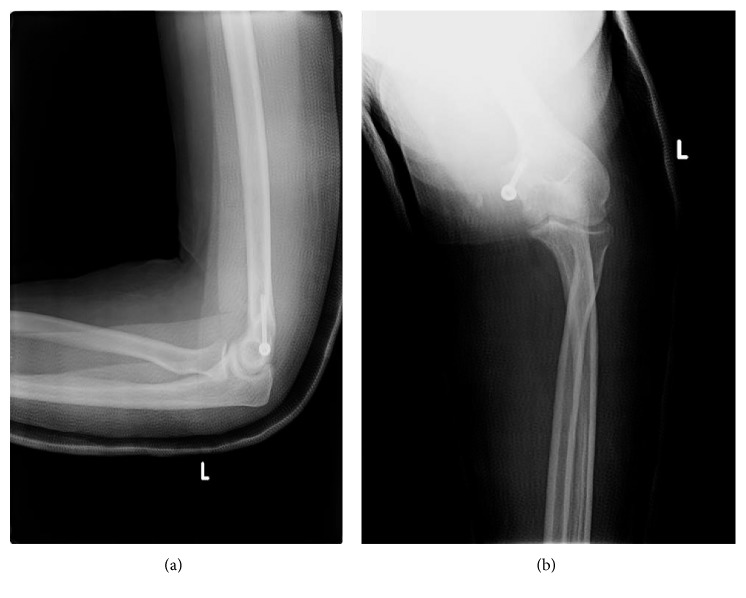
Postoperative control X-rays showing good reduction and compression of the medial epicondyle fragment. To note, the patient has an above-the-elbow posterior cast splint.
